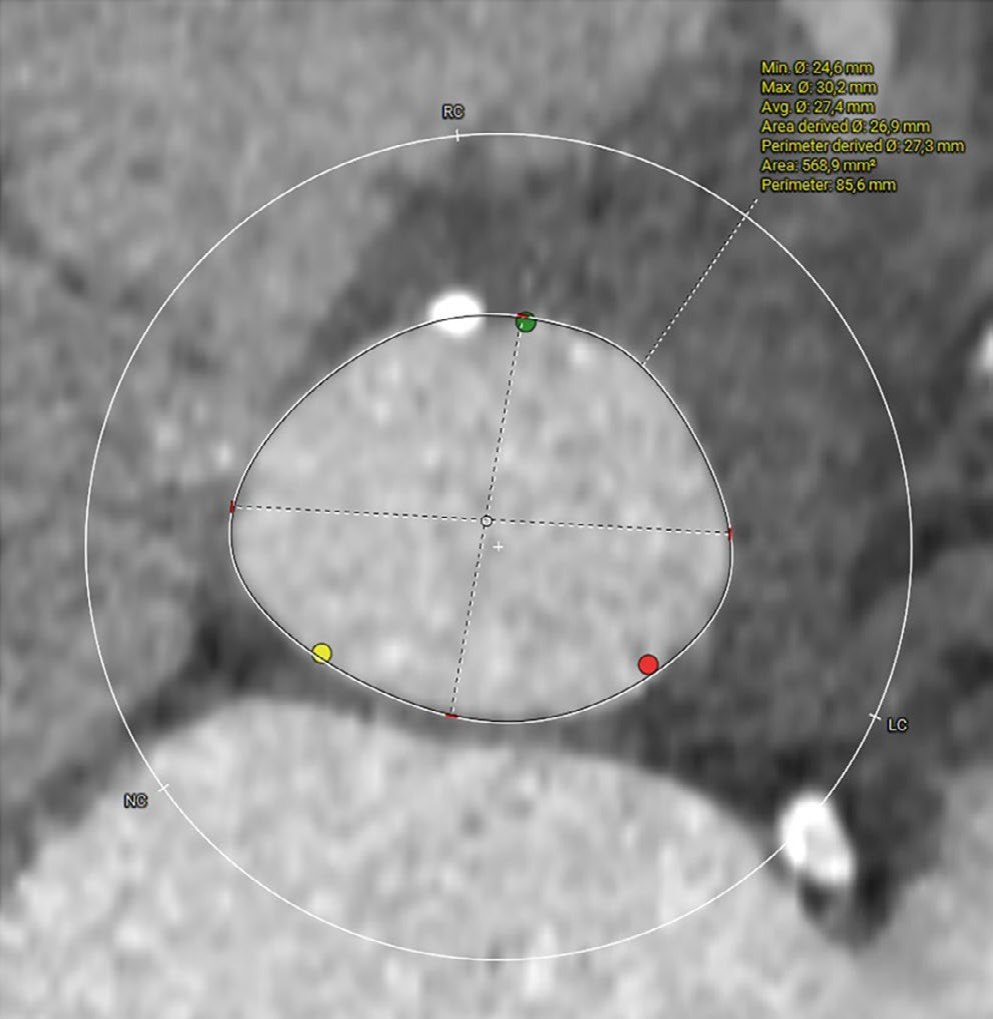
Measurement of the aortic ring size and prosthesis selection based on the pre‐TAVI CT scan.
